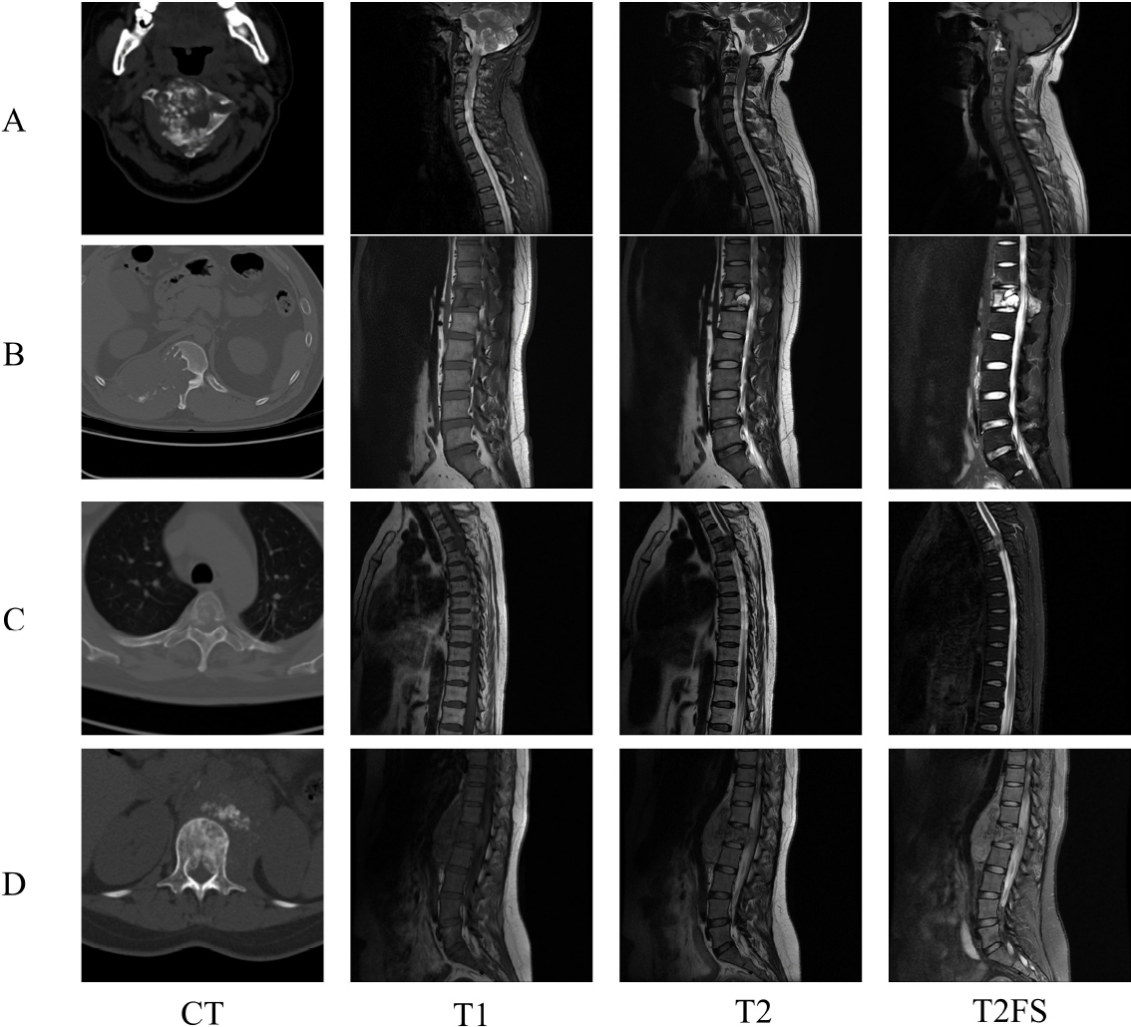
Controversial cases of osteosarcoma and chondrosarcoma. a, b: Cases where radiologists misdiagnosed osteosarcoma as chondrosarcoma; c, d: Cases where radiologists misdiagnosed chondrosarcoma as osteosarcoma. Notably, the radiomics model achieved correct diagnoses in all cases. T2FS, T2-weighted fat-suppressed.
